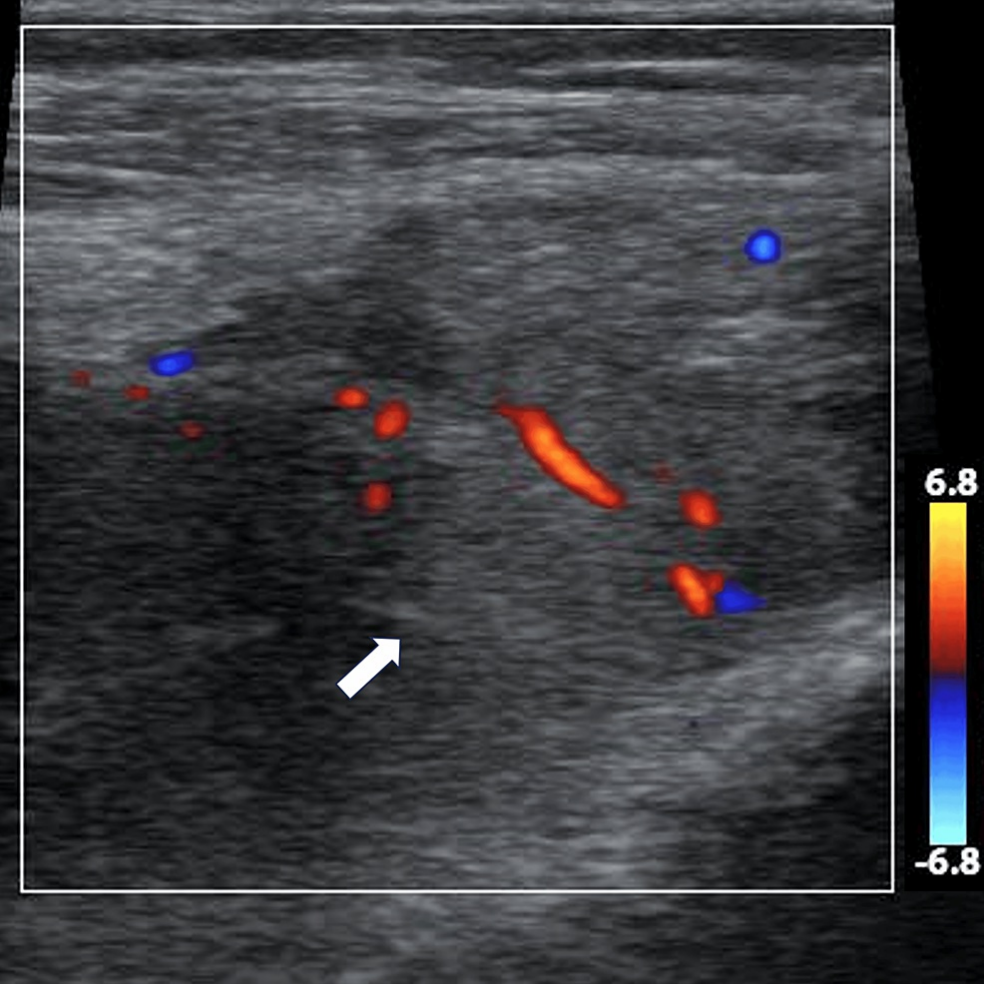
Ultrasound image of suprapatellar synovial hypertrophy with Color Doppler highlighting areas of hypervascularization
